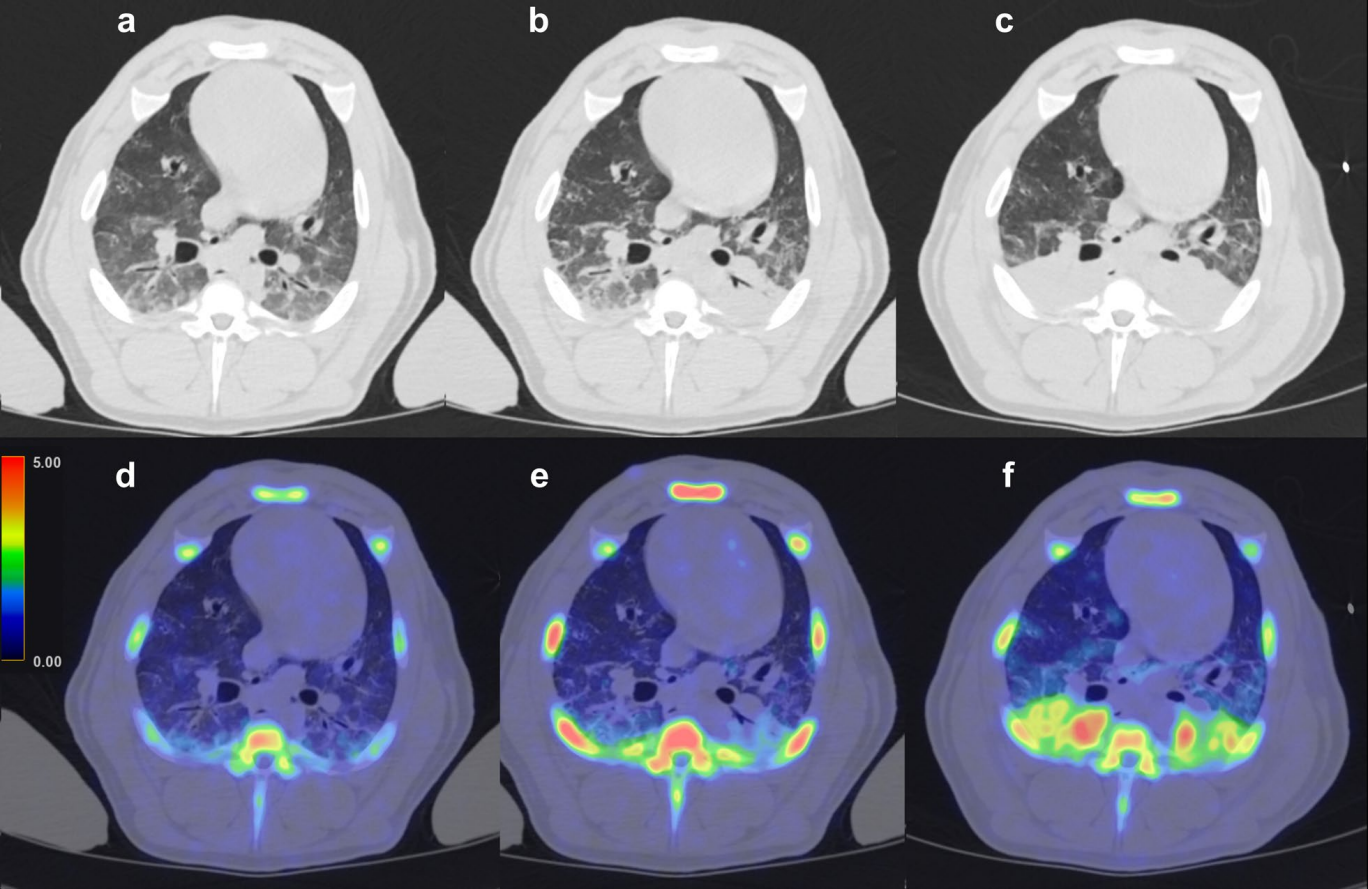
Upper row: trans-axial CT-scans images over the lungs: a − 1 h, b 2 h, c 4 h. Lower row: corresponding trans-axial fused PET–CT images with [^11^C]NES. d − 1 h, e 2 h, f 4 h. All images are corrected to SUV = 5 and thus directly comparable. The amount of NE in the lungs increases as the sepsis progresses
